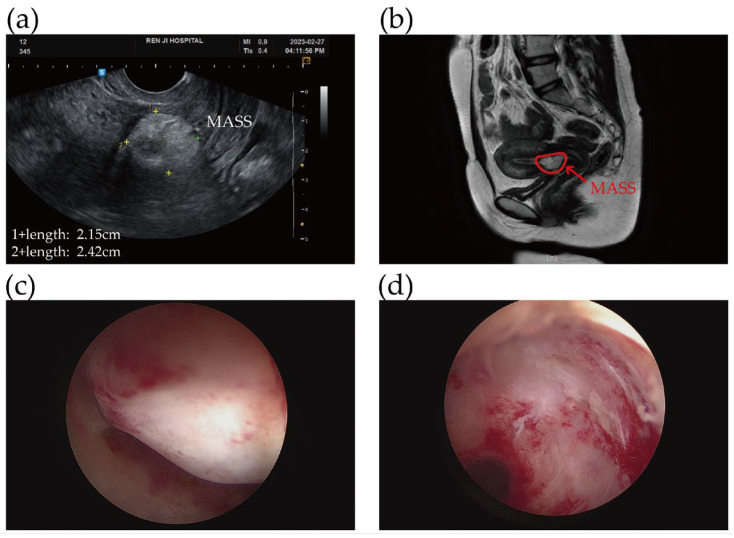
(a) Transvaginal ultrasound image showed that an isoechoic echo tumor measuring approximately 2.15 × 2.42 cm, originated from the lower uterine segment extending to cervix. (b) MRI study suggested an intracavitary lipid-based teratoma measuring 2.4 cm. (c) A myoma-like mass was seen in the lower uterine segment near the internal cervical os by hysteroscopy. (d) The tumor was completely enucleated after the IBS^®^ treatment.
